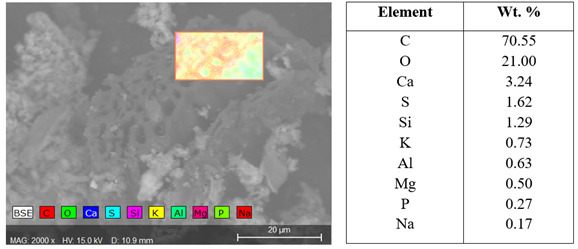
SEM-EDX map for all elements distribution across the area highlighted in image and associated energy dispersive X-ray (EDX) quantification of biochar.
